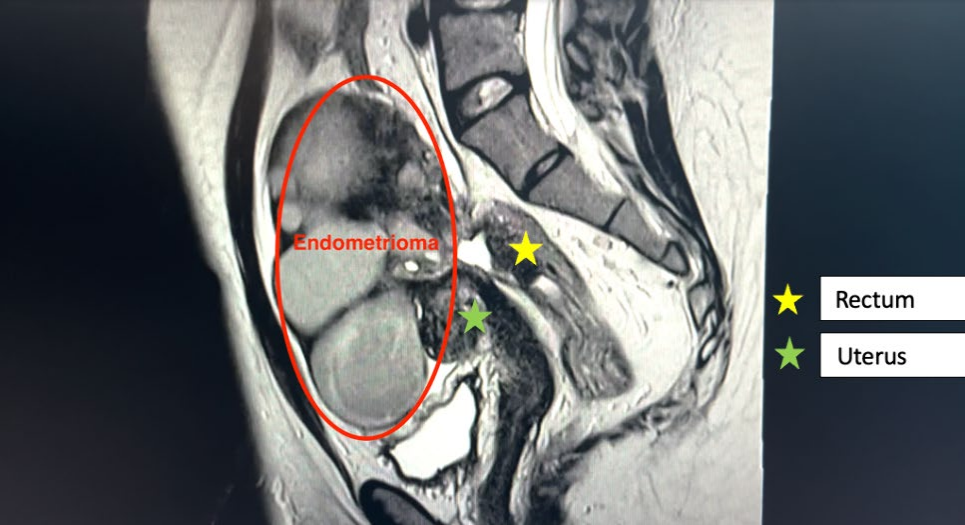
The preoperative MRI showed a 13-cm endometriosis conglomerate incorporating both ovaries, the outer uterine wall, and the anterior rectosigmoid
